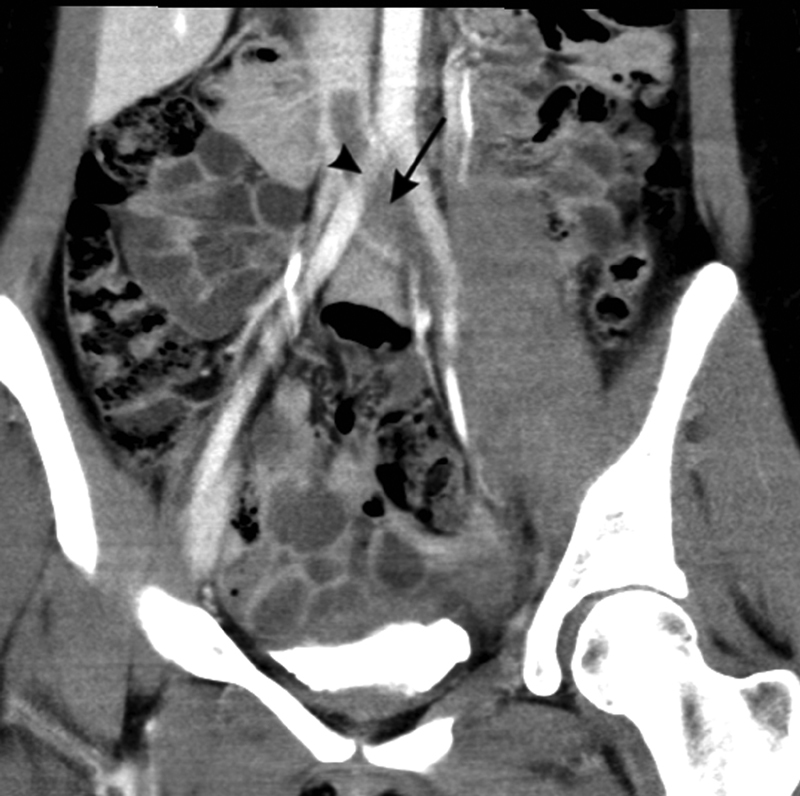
Postcontrast sagittal view of CT of abdomen/pelvis demonstrating thrombus within left common iliac vein (
